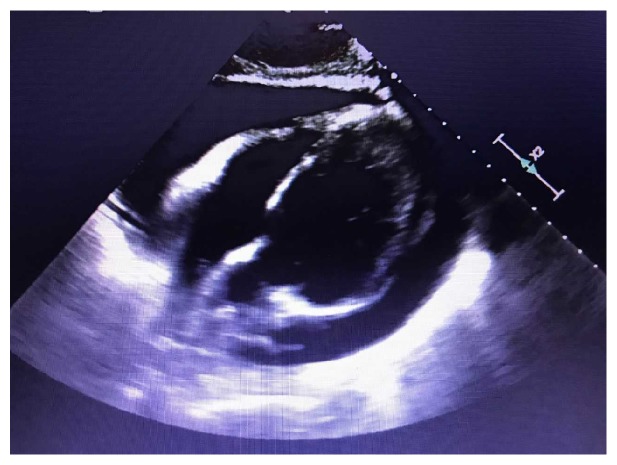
Echocardiogram prior to pericardial drain placement showing large pericardial effusion.
